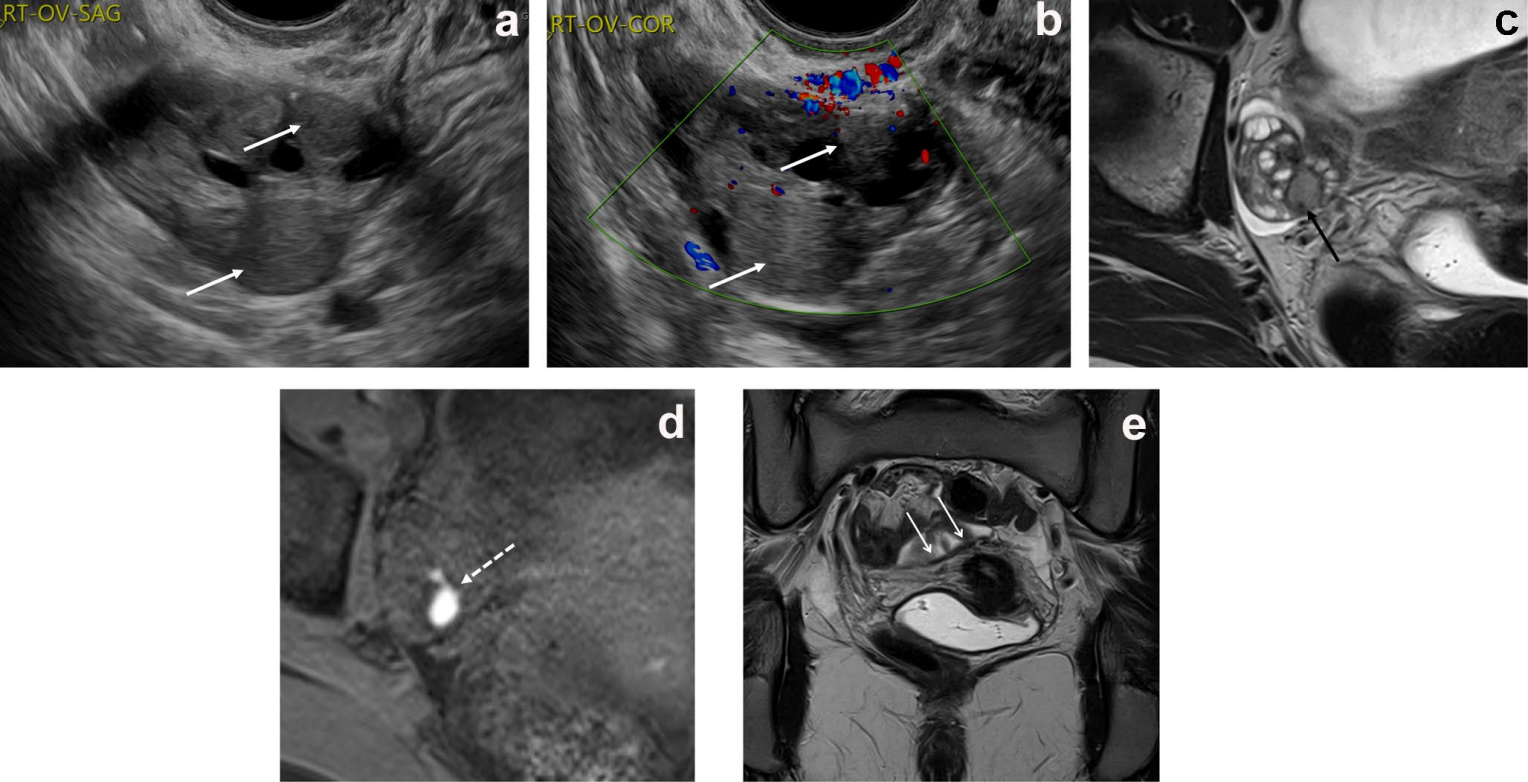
Images from a 19-year-old patient with chronic pelvic pain, dyspareunia, and a family history of endometriosis. a Transvaginal ultrasound image through the right ovary shows 2 small cystic structures with low-level internal echoes characteristic of endometriomas (white arrows with closed arrowheads). b Image through the right ovary with color Doppler shows no vascular flow within the lesions (white arrows with closed arrowheads). Pelvic MRI was performed 9 months later. c T2W axial image shows one of the 2 endometriomas with T2 shading and a dark spot sign (black arrow). d The lesion shows characteristic homogeneous hyperintense signal on the corresponding axial T1W image (dashed arrow). e Coronal T2W image through the torus uterinus/uterosacral ligaments shows asymmetric, irregular thickening of the right uterosacral ligament (white arrows with open arrowheads). Oral contraception was initiated after imaging
